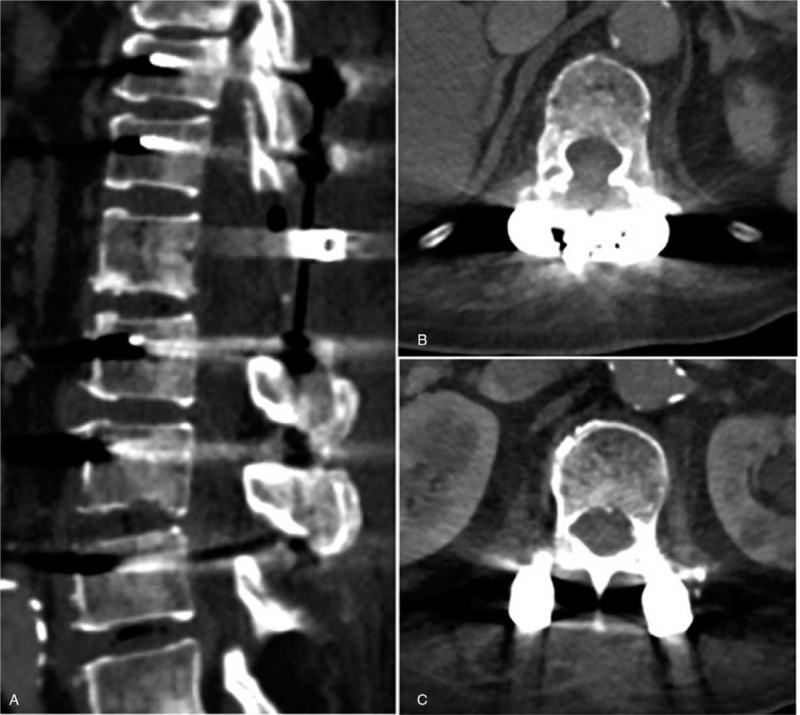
Postoperative computed tomography scan showing improved air density at epidural space (A) and significantly decreased air density at T12 (B) and L1 (C) vertebral bodies.
